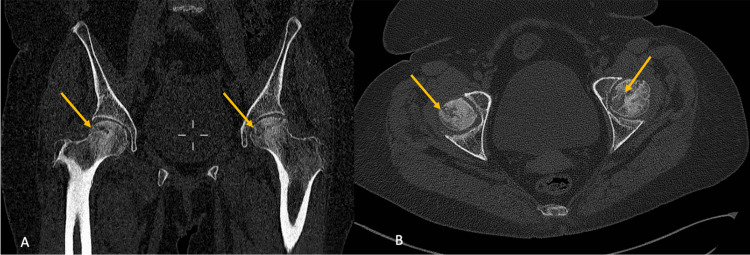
CT of the pelvis bone window: (A) Coronal section and (B) axial section show the alteration of the asterisk by loss of its rays and by peripheral increase of the density which translates into extensive necrosis of the femoral head stage 3 according to Ficat for the left femoral head and stage 2 for the right one.
